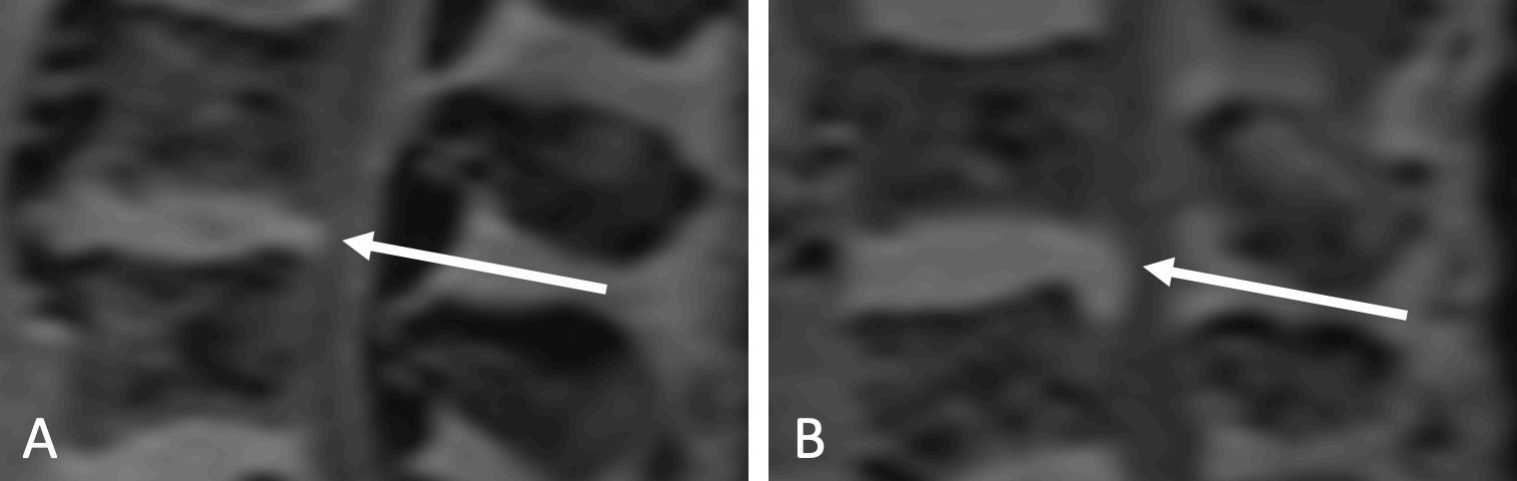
Image example of disc bulging (A) and disc protrusion (B) on L3/4 as indicated by the arrows (MRI sequence: VIBE Dixon Iso W).
